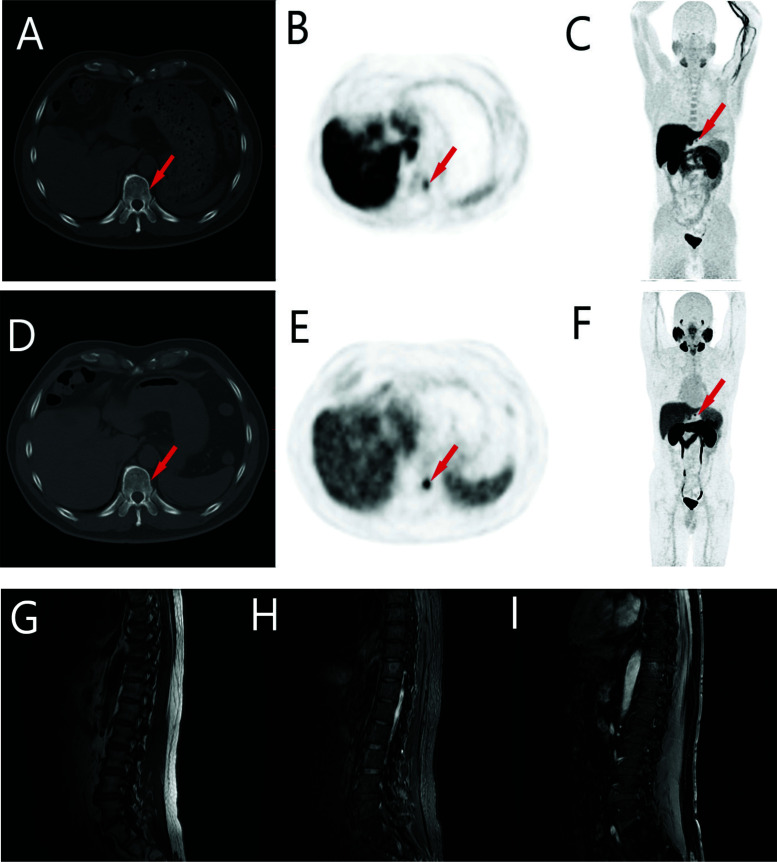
Comparison of PET imaging using [11C]CHO and [18F]MD-PSMA in the same patient in 09/2017. The lesion is shown by arrows. The 1^st^ row (A-C) has the PET images using [11C]CHO, and the 2^nd^ row (D-F) has the PET images using [18F]MD-PSMA. From left to right, the images are CT-cross-section, PET cross-section, and maximum intensity projection (MIP) map, respectively. The 3^rd^ row (G-I) has contemporaneous MRI images, demonstrating metastasis. From left to right, the images are T1-weighted. T2-weighted, and T1-enhanced, respectively.
